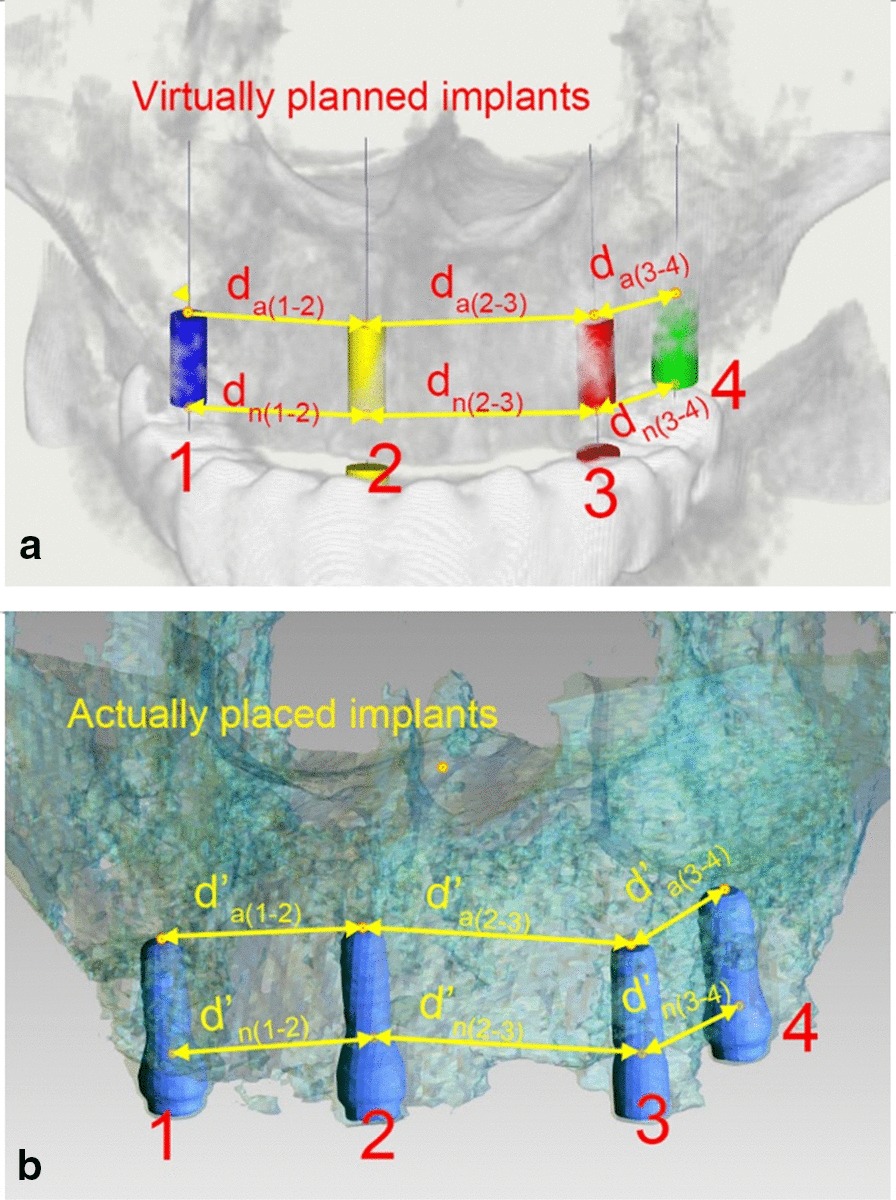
a, The inter-implant distance at the neck level of two neighbouring implants in the virtual planning model was named d_n_, and that at the apex level was named d_a_; b, The inter-implant distance at the neck level of two neighbouring implants in the post-operative CBCT scan was named d_n_′, and that at the apex level was named d_a_′; The mean difference between the virtual and actual inter-implant distances of two adjacent implants was calculated
